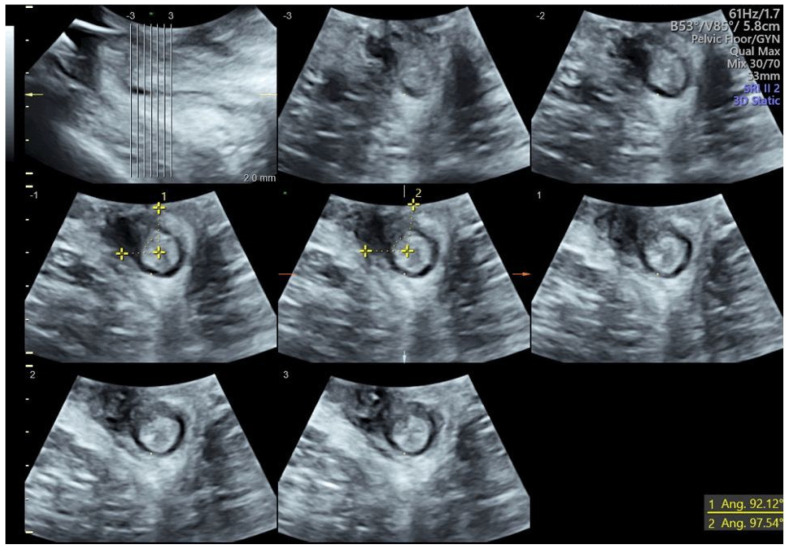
Three-dimensional transperineal ultrasound performed before secondary repair. The angle highlights the external anal sphincter injury at the 11 o’clock position.
